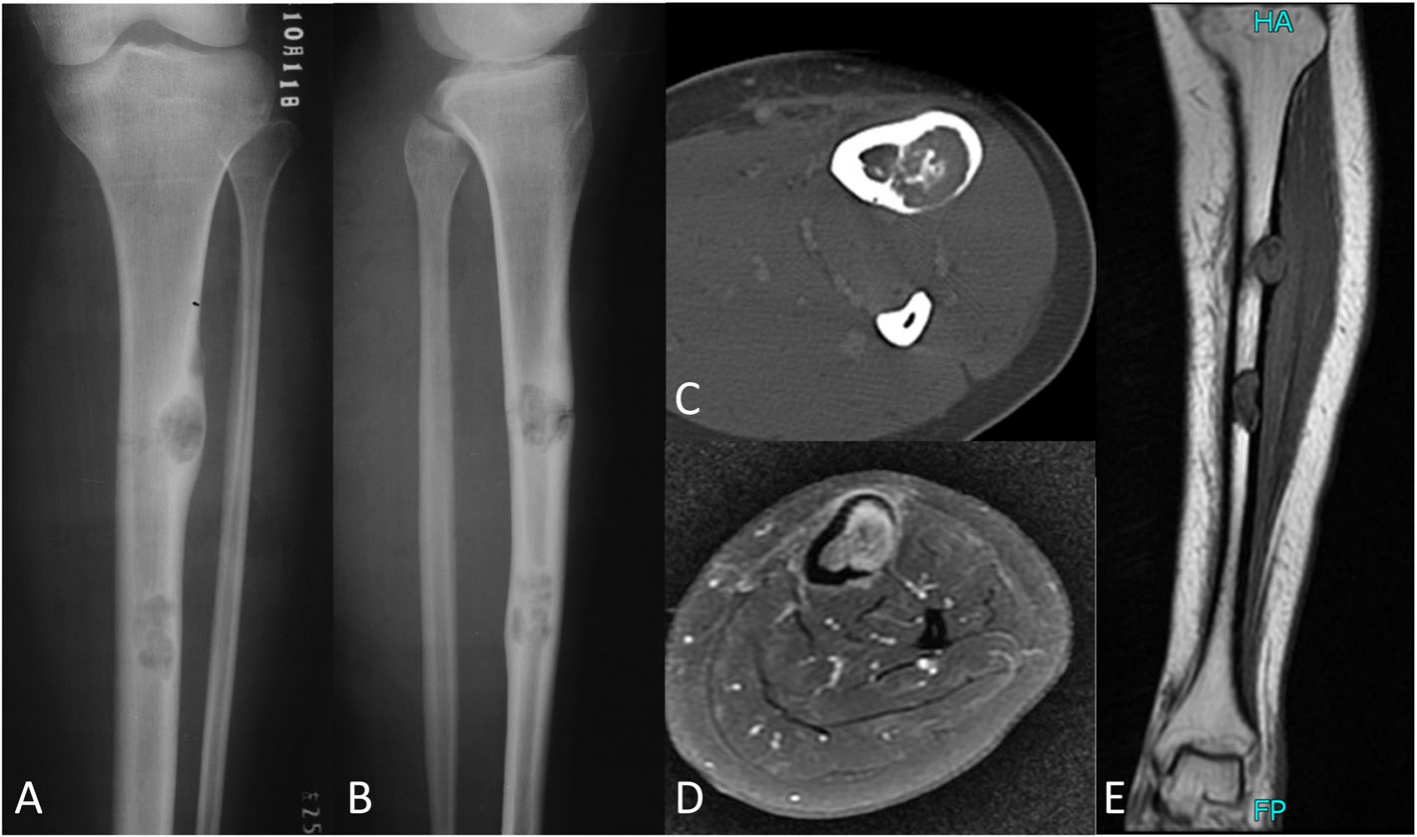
Representative images of classic adamantinoma. Radiographs showing a anterior-posterior and b lateral views of two lesions in the tibial shaft. c CT image showing the extent of the tumor in the cortex. d, e MR images showing the extent of the tumor
